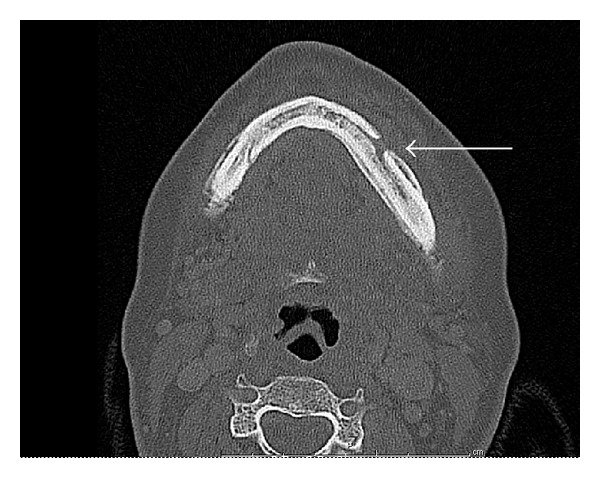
Repeat CT scan of the mandible. Repeat CT scan of the mandible showed changes along the external and internal surface of the mandible (arrow) with production of new bone along the body extending to the mandibular angle thought to be secondary to osteonecrosis from the radiation therapy.
